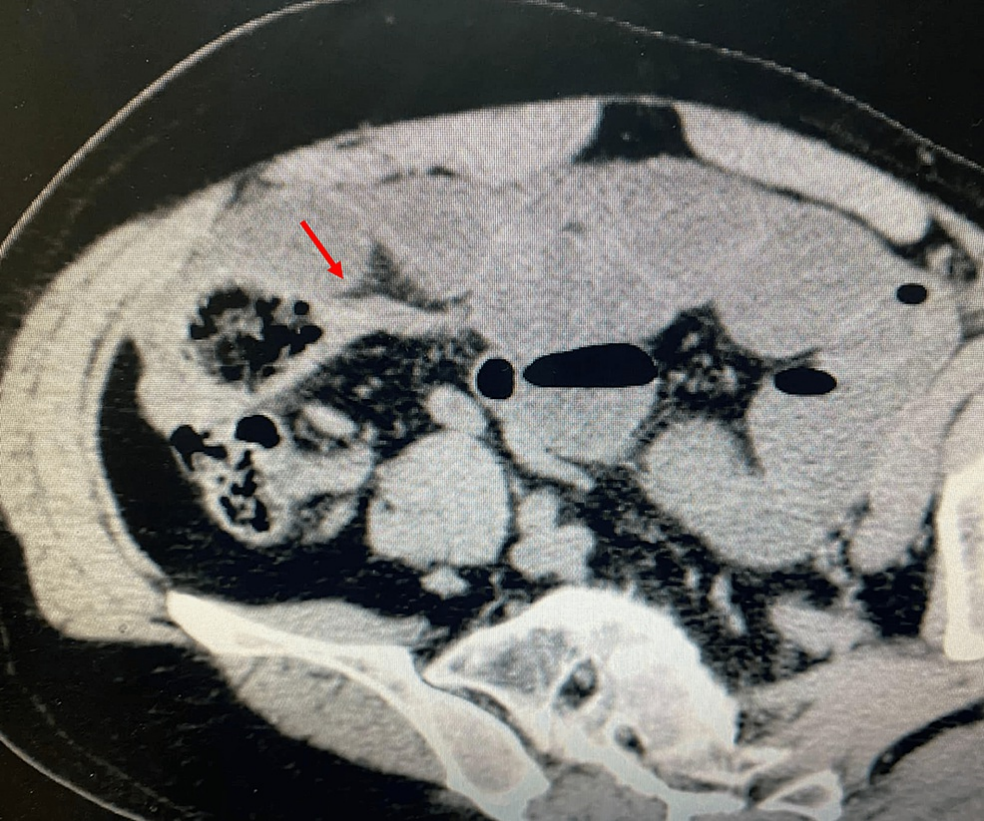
An axial cut of an abdominal CT scan also showed a transition zone in the small bowel (red arrow).
